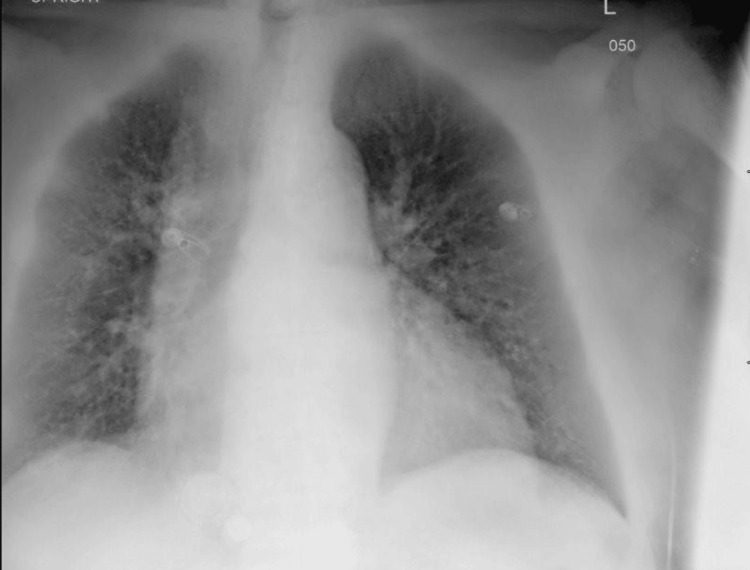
Chest X-ray showing pulmonary vascular congestion
